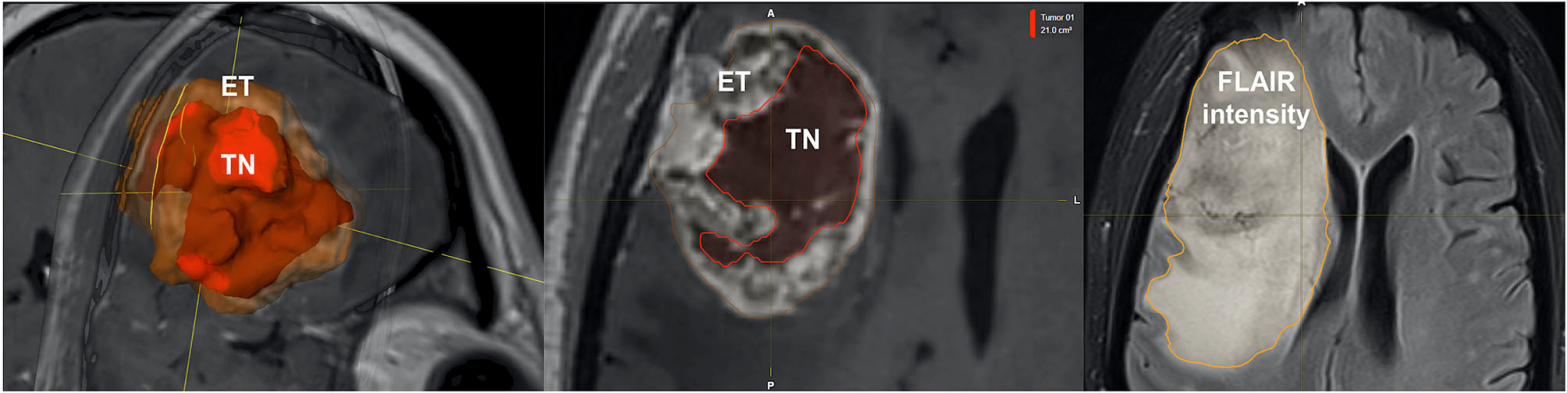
Representative volumetric parameters calculated by the semi-automatic method. Similar compartments were measured by the 2D and, 3D ellipsoid methods. Left: Enhancing tumor (ET), Center: Tumor necrosis (TN), Right: Fluid-attenuation inversion recovery (FLAIR intensity).
